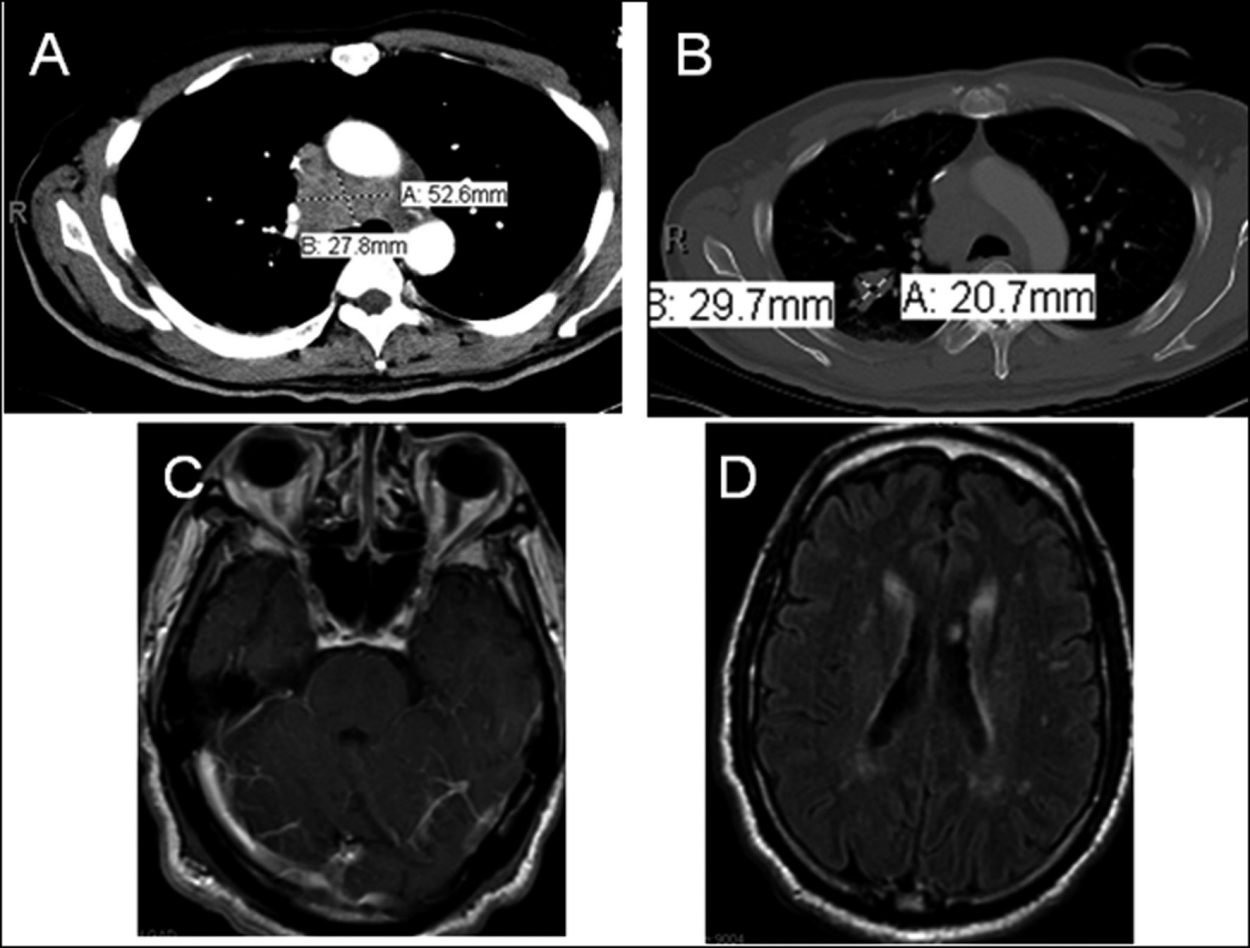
CT Chest demonstrating mediastinal mass (A) and right lung parenchymal lesion (B). Brain MRI T1 post-gad (C) showing cerebellar enhancement, FLAIR image (D) indicating diffuse white matter signal changes.
